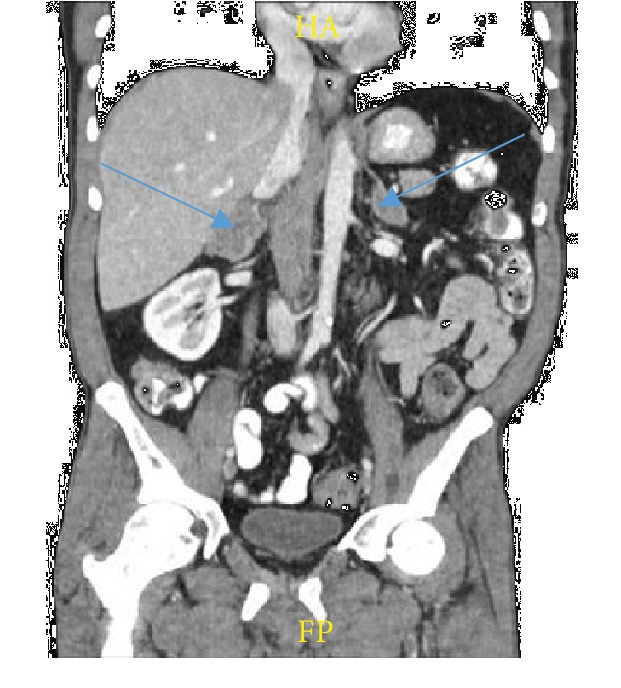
CT abdomen and pelvis with IV and PO contrast showing the right adrenal mass increased from 3.4 × 2.0 cm to 4.3 × 2.9 cm with more irregular margins. The left adrenal mass increased from 2.9 × 1.6 cm to 3.9 × 2.0 cm. Arrows pointing to adrenal masses.
